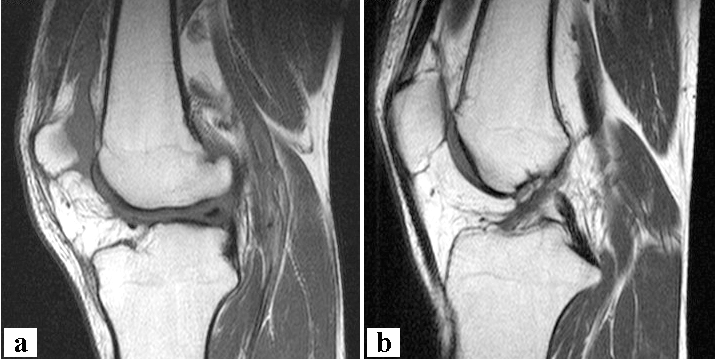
Magnetic resonance imaging. Sagittal T1 TSE sequences of the affected (a) and the contralateral (b) knee. Note the lack of both cruciate ligaments and the abnormal tibial eminence.
